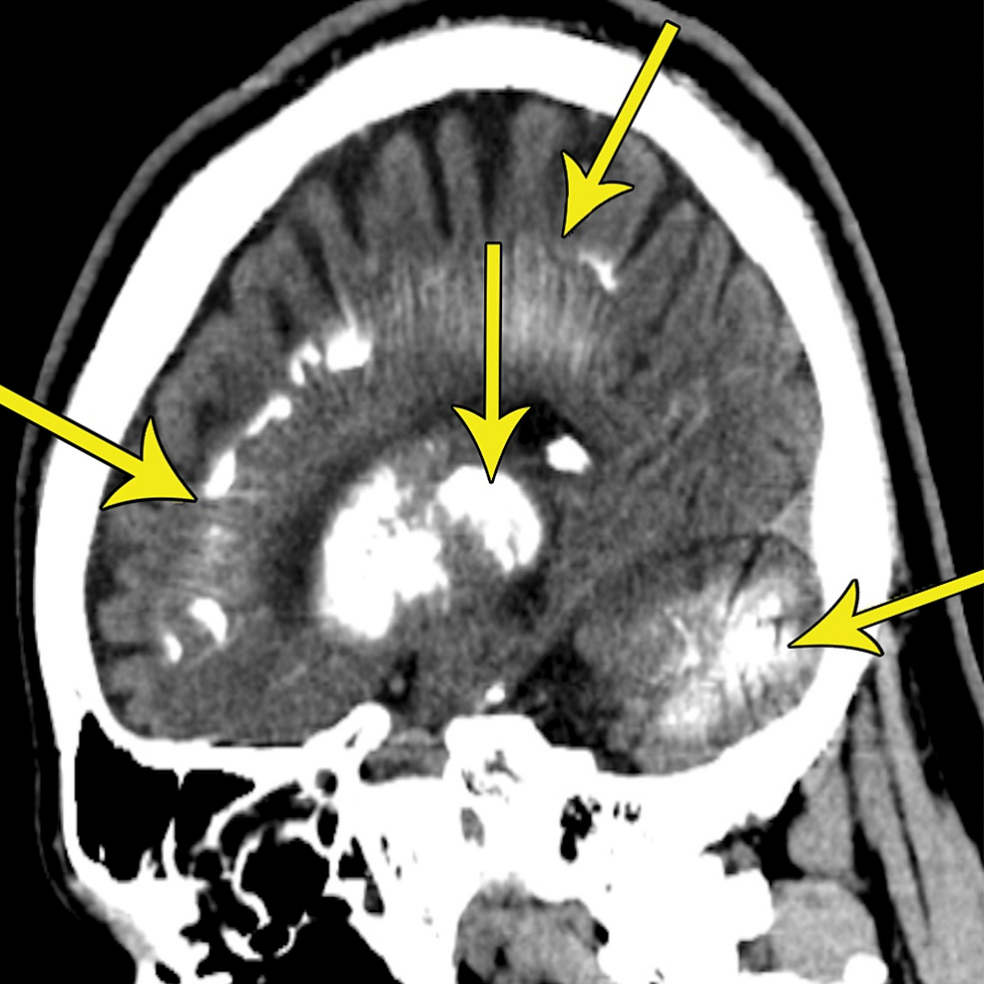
Sagittal non-contrast CT demonstrates extensive symmetric calcification of the subcortical gray-white junctions, basal ganglia, thalami, cerebellar nuclei, and corona radiata (yellow arrows) CT: computed tomography
